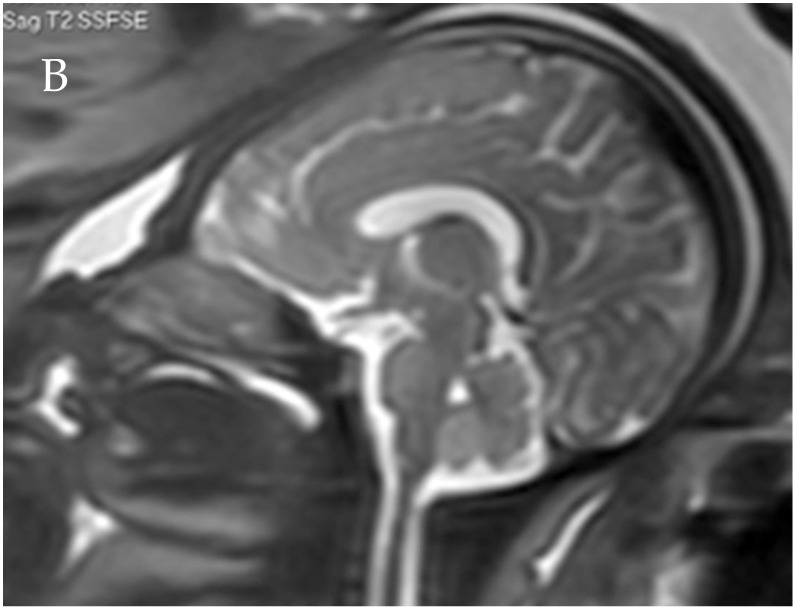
Corpus callosum as captured by trans-abdominal mid-sagittal US scan at 23 weeks of gestation (A) and by mid-sagittal MRI scan at 33 weeks of gestation (B).
